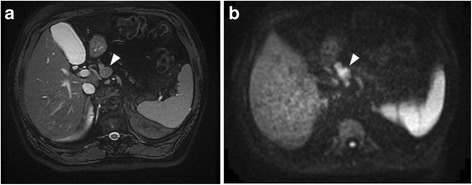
Magnetic resonance imaging of the abdomen. a T2-weighted image showing a clear, round, mass in which the intensity was lower than the intensity of water. b Diffusion-weighted image showing a high-intensity mass (*arrowheads*)
